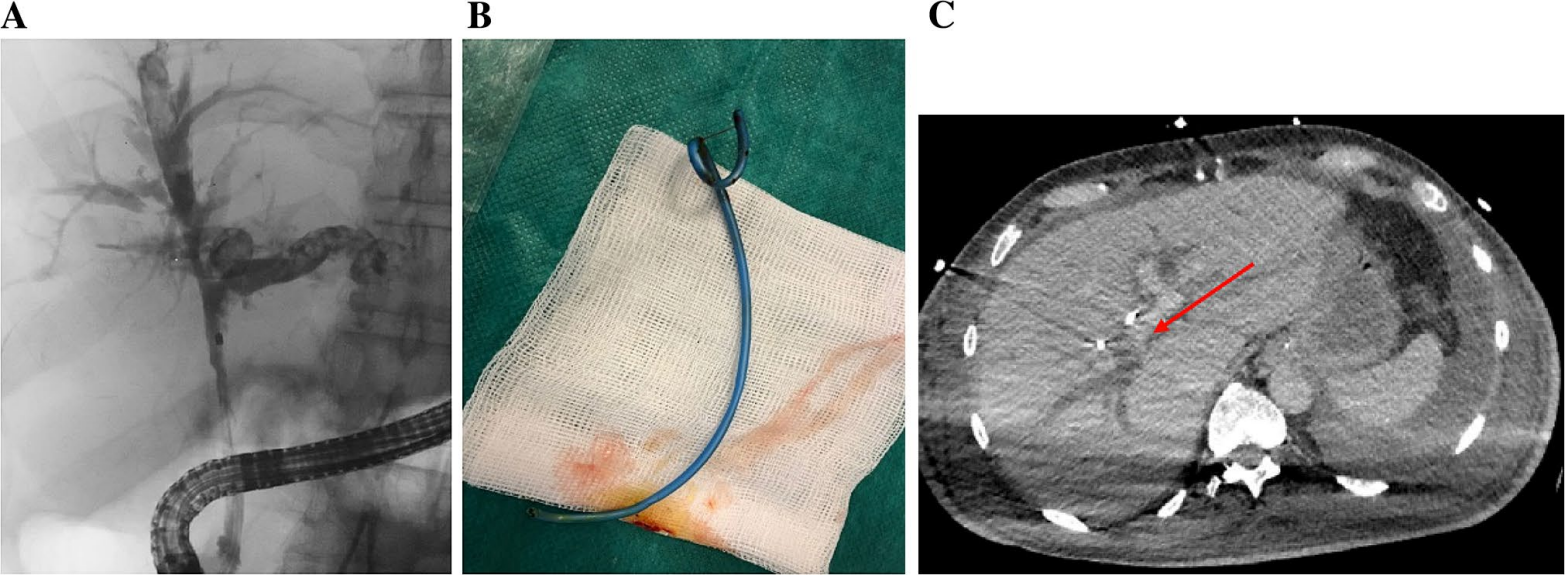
a ERCP of the bile duct system with cholangiocarcinoma. b Extracted 7 French bile duct stent. c CT-scan with cholangiocarcinoma (arrow)
